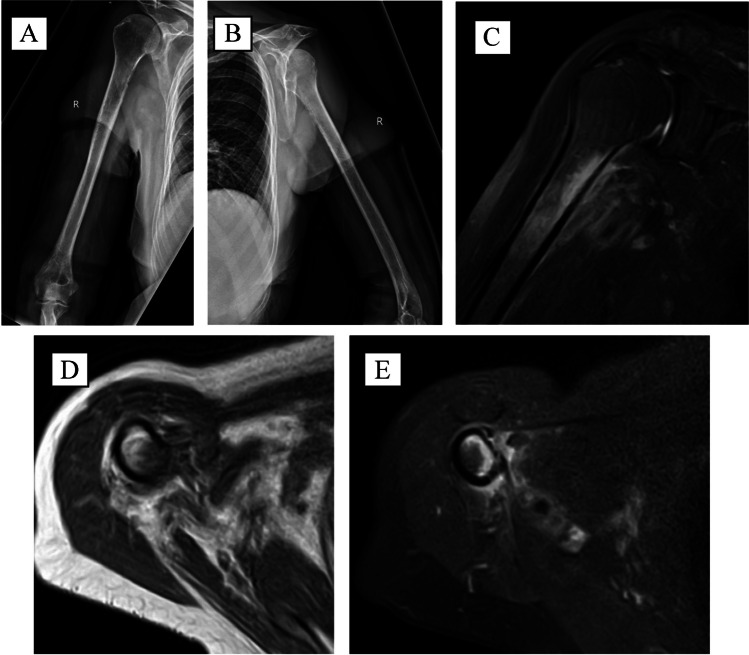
4/2021 — AP (A) and lateral (B) right humerus radiographs demonstrating an infiltrative lesion involving the proximal diaphysis with medial cortical erosion. Coronal T2 FS FSE (C), axial T1 (D), and T2 FS FSE (E) MRI sequences showing erosion of the medial cortex at the level of the proximal diaphysis with surrounding intramedullary and extramedullary edema
